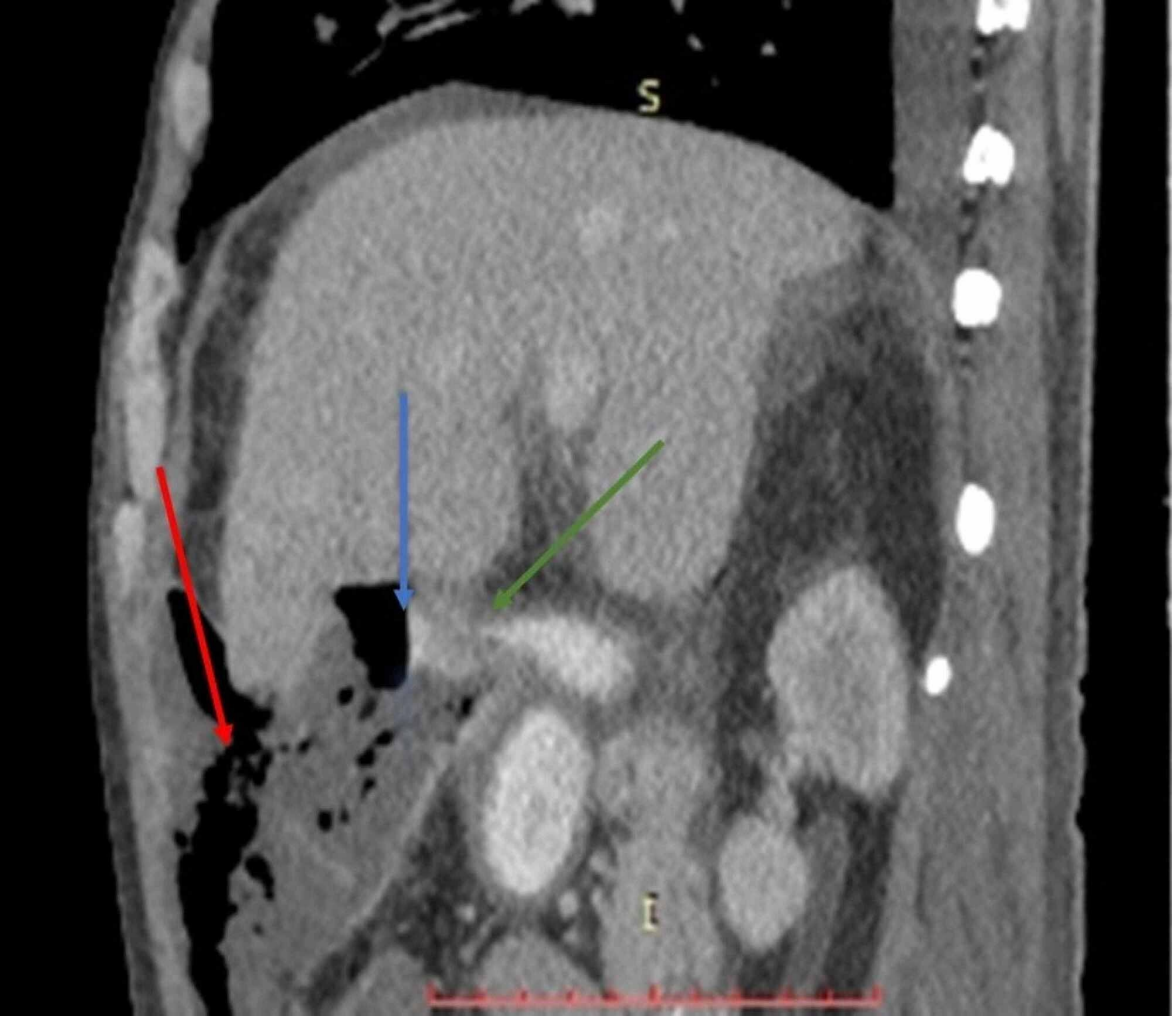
Sagittal reconstruction of contrast-enhanced CT of upper abdomen showing a contrast filled fistulous tract connecting the air containing liver abscess and first part of duodenum (green arrow). Note the contrast fluid level within abscess (blue arrow) and anterior rupture of abscess in pre-peritoneal space (red arrow).
